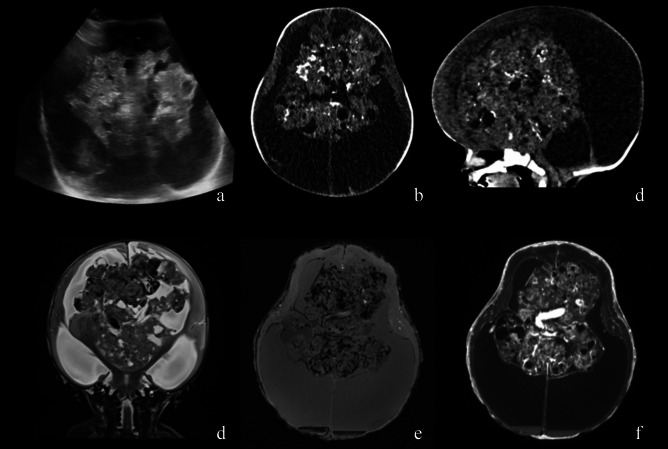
Mature teratoma*. Neonatal US (a), CT (b, c) and MRI (d–f) demonstrating a heterogeneous midline intraventricular mass containing calcification, fat and solid components causing severe mass effect with hydrocephalus and diffuse brain edema. Prominent vascular structures are also evident within the lesion. Brain tissue is severely compressed and displaced at the periphery. Brain sulci and basal cisterns are partially effaced
